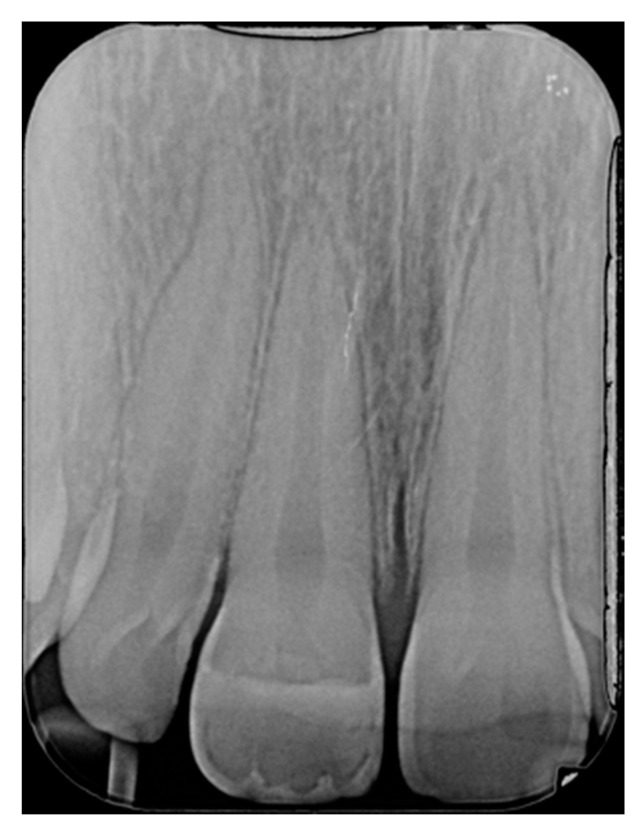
One year post-operative x-ray. Reprinted from Restauri diretti nei settori anteriori, G. Paolone, S. Scolavino, © 2021, with permission from Quintessence Publishing Italy.
